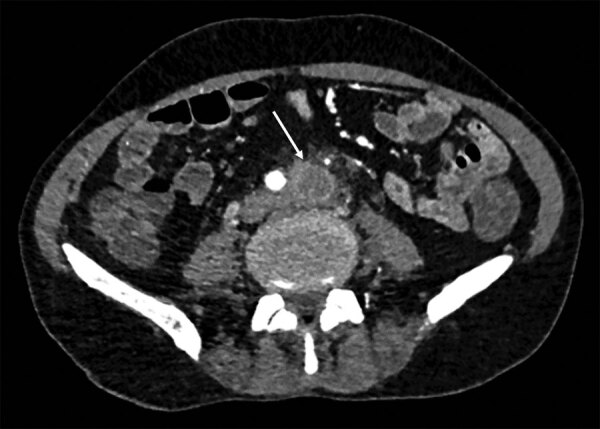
Abdominal computed tomography scan for case-patient 1 showing arteritis in study of unexpected vascular locations of *Scedosporium* spp. and *Lomentospora prolificans* fungal infections, France. Arrow indicates primitive left iliac artery thrombosis and perivascular soft tissue contrast. Data are from the Scedosporiosis/lomentosporiosis Observational Study (*12*).
